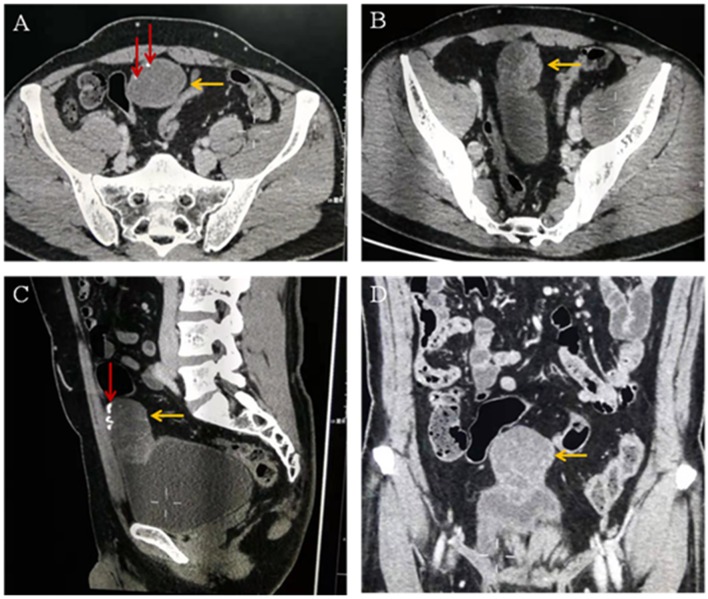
(A–C) Computed tomographic images showing a cystic solid mass (yellow arrow) in the anterior part of the bladder measuring 6.8 × 3.9 cm in size. The tumor invades the anterior wall and peritoneum of the adjacent bladder. Calcification (red arrow) and separation (yellow arrow) are visible within the tumor. (D) Enhanced computed tomographic image showing that the solid part of the mass is obviously enhanced (yellow arrow).
